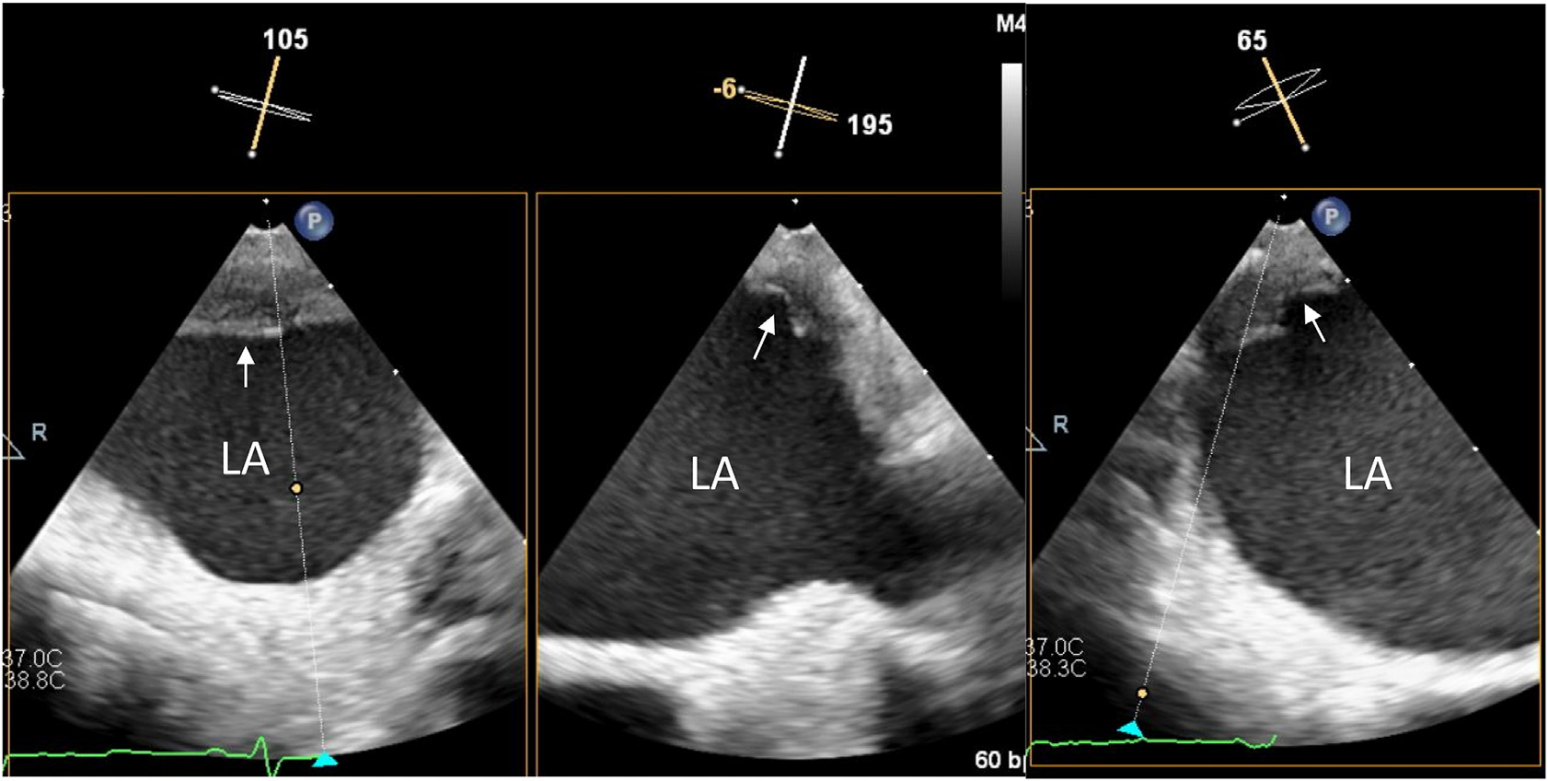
Transesophageal echocardiography done just after termination of the procedure. The posterior wall thrombus was documented in the views not directed by guideline (*arrows*).
